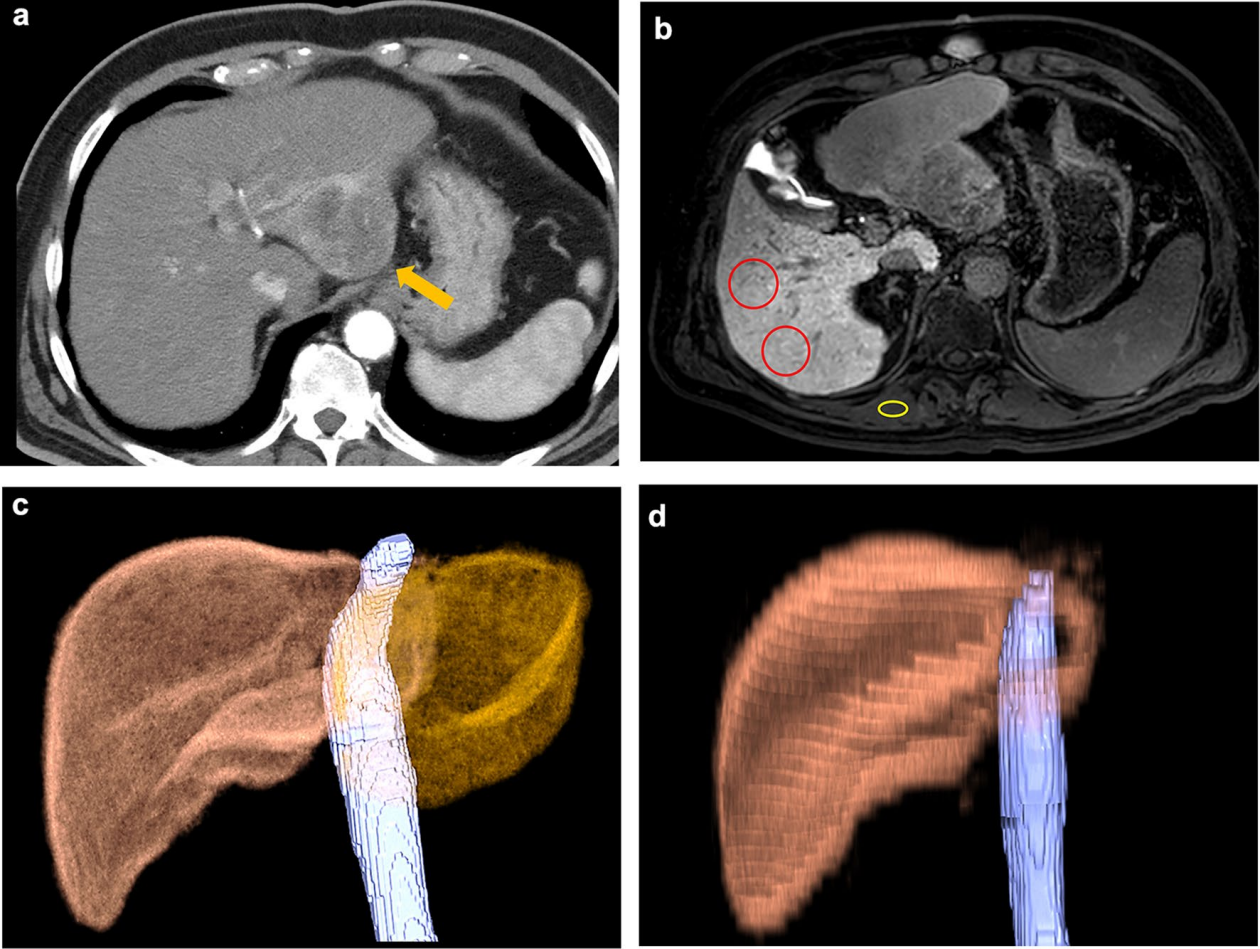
A 79-year-old man with hepatocellular carcinoma in the left lobe of the liver. a Arterial-dominant phase of dynamic CT shows heterogeneously enhanced mass in the left lateral segment of the liver (arrow). b The hepatobiliary phase of gadoxetic acid-enhanced MRI. ΔLMR of the right lobe was 0.33. c Preoperative CT volumetry. The right and total liver volumes were 620 and 944 mm^3^, respectively. The RR was 34.3%. d Postoperative CT volumetry performed 86 days after the left hemihepatectomy. The right liver volume was 913 mm^3^ and the LRI was 47.3%
